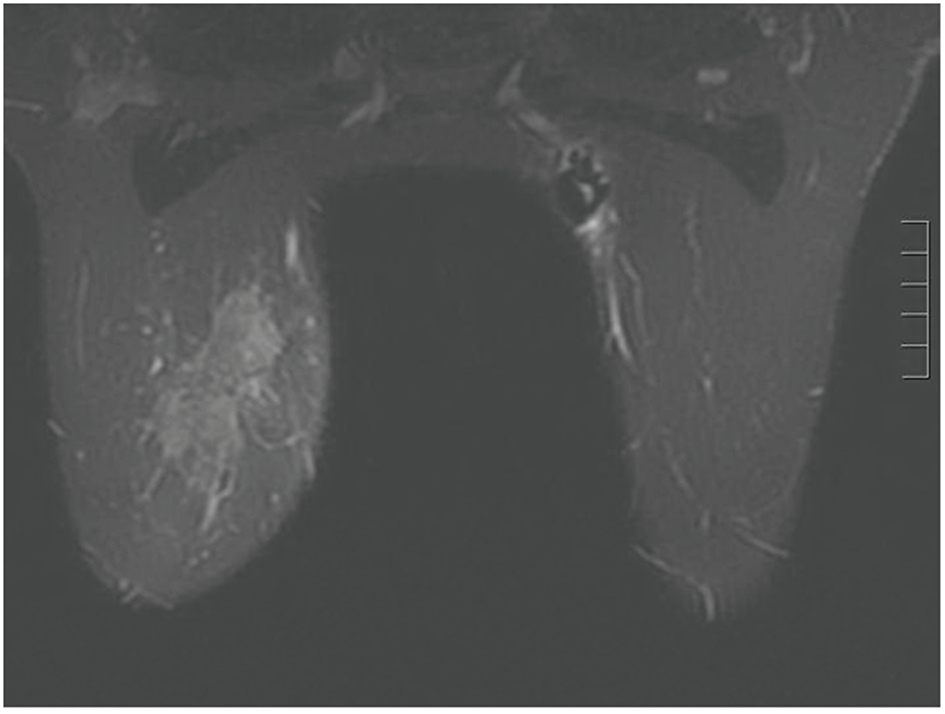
Breast MRI 2012. Given the large triple negative cancer, a breast MRI and PET-CT scan were performed for staging purposes. The PET-CT showed a 5 cm left breast mass which was FDG-avid, as well as an 8 mm left axillary LN and a 5 - 6 mm left internal mammary node both also FDG-avid. No distant metastasis was evident. The left axillary LN underwent an ultrasound-guided biopsy that proved metastatic from the breast. The breast MRI showed a 7.1 × 3.3 cm enhancing mass at the left breast 10:00 - 11:00 position, and a 2.8 cm axillary LN, both of which are already biopsy-proven malignancies. After workup, the patient’s final clinical stage was cT3 N3 M0 - stage IIIC. A multidisciplinary breast conference was held and the group decided that treatment should include neoadjuvant chemotherapy, surgery, whole breast RT including regional basins, and genetic counseling for BRCA testing. The patient underwent six cycles of TAC (docetaxel, doxorubicin and cyclophosphamide) which she finished in January 2013. BRCA testing revealed no mutation in the BRCA 1 and 2 genes. The post-chemotherapy physical exam showed excellent clinical response with no palpable evidence of disease in the left breast. Breast MRI and PET-CT (February 2013) both also showed complete clinical response (both breast tumor and lymphadenopathy). Brain MRI also showed negative findings.
